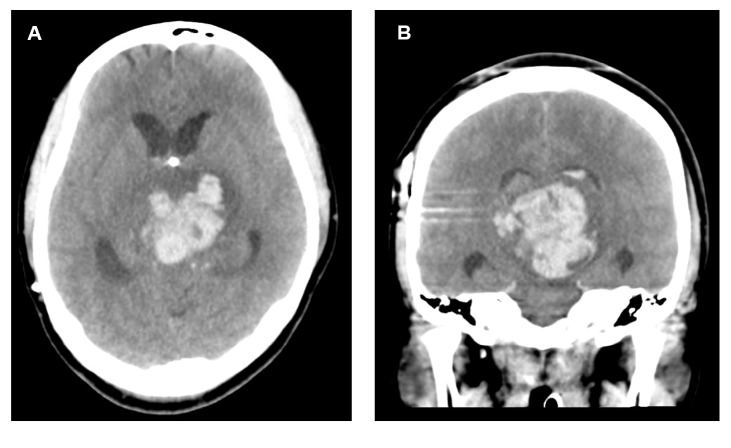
The emergency head CT on postpartum day 2 revealed cerebral herniation due to massive intratumoral hemorrhage ((A); axial view, (B); coronal view).
